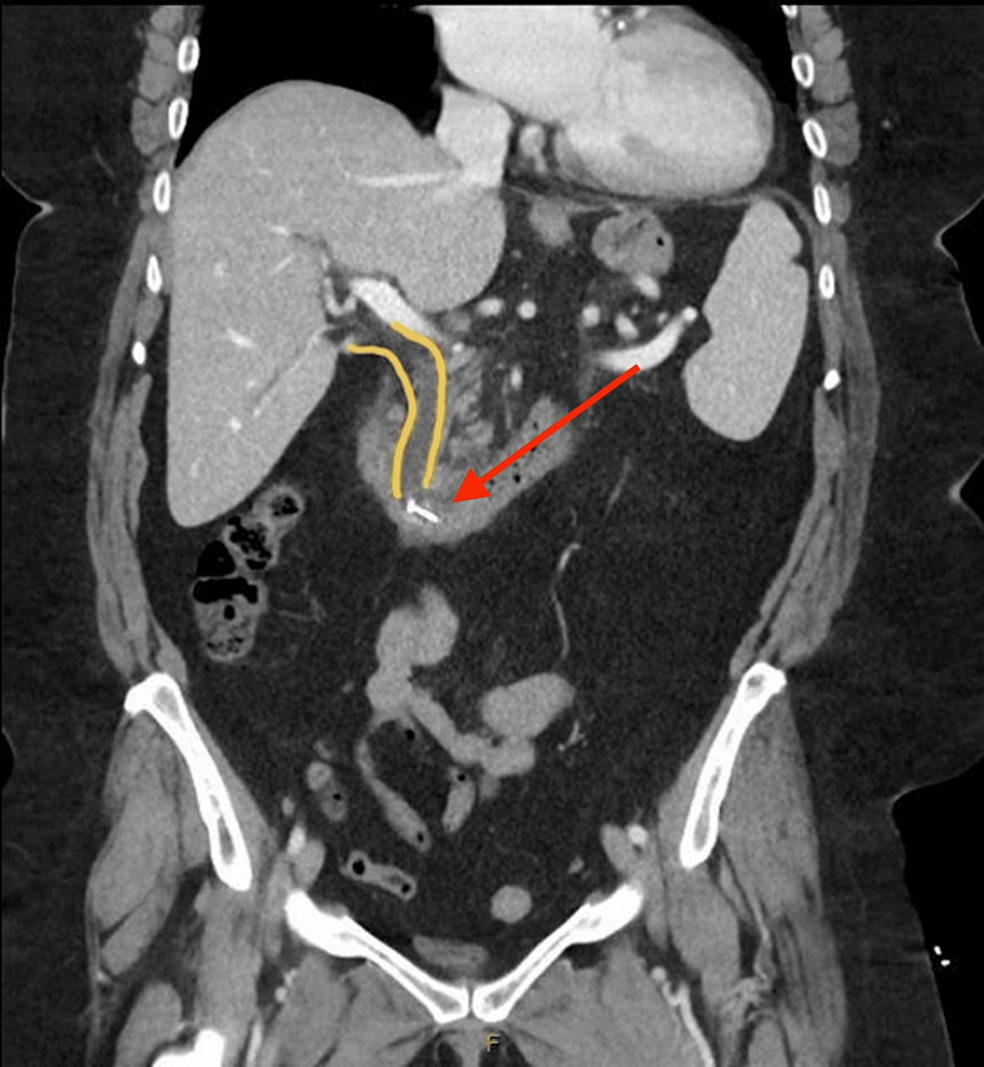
CT imaging of the patient's second presentation demonstrating a dilated common bile duct (CBD) as indicated by yellow lines with a migrated radio-opaque clip noted within the CBD, as shown by the red arrow
